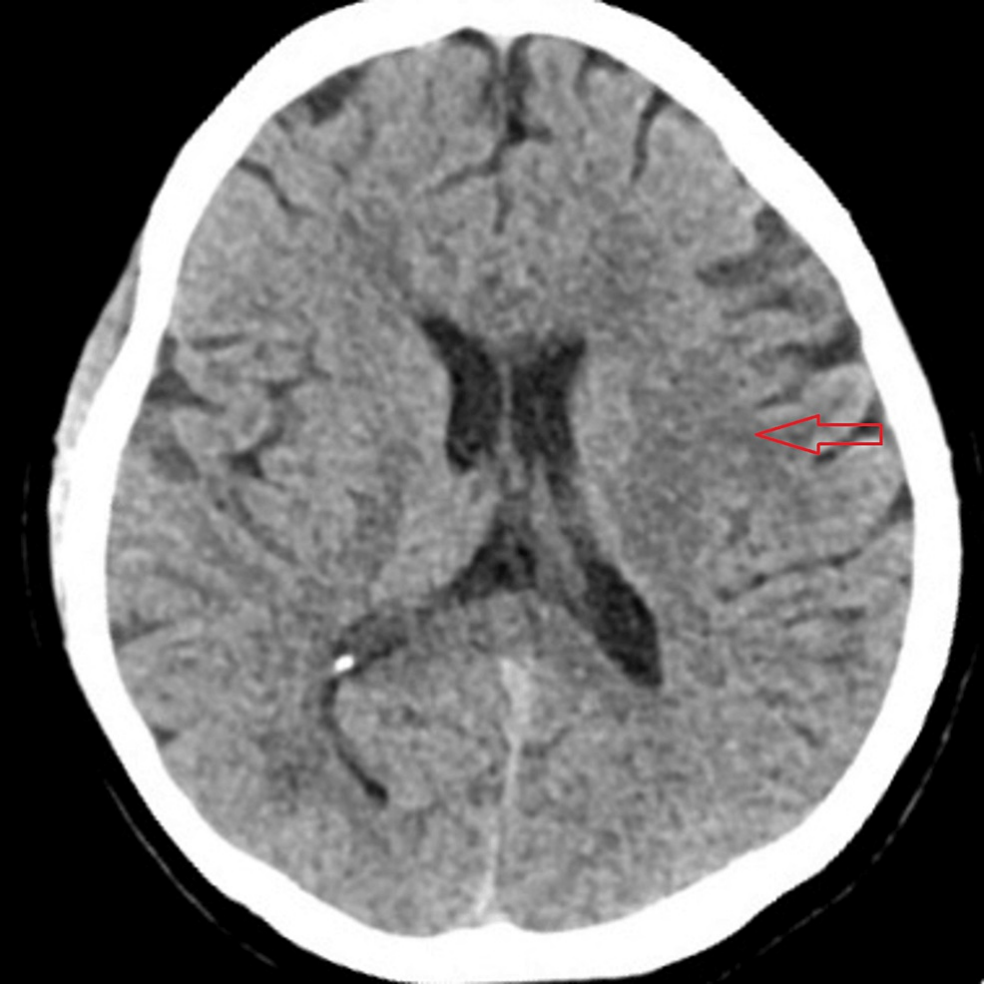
Computed tomography (CT) of the brain of Case 4 CT brain of Case 4 showing a lacunar infarction of the left globus pallidus (red arrow)
